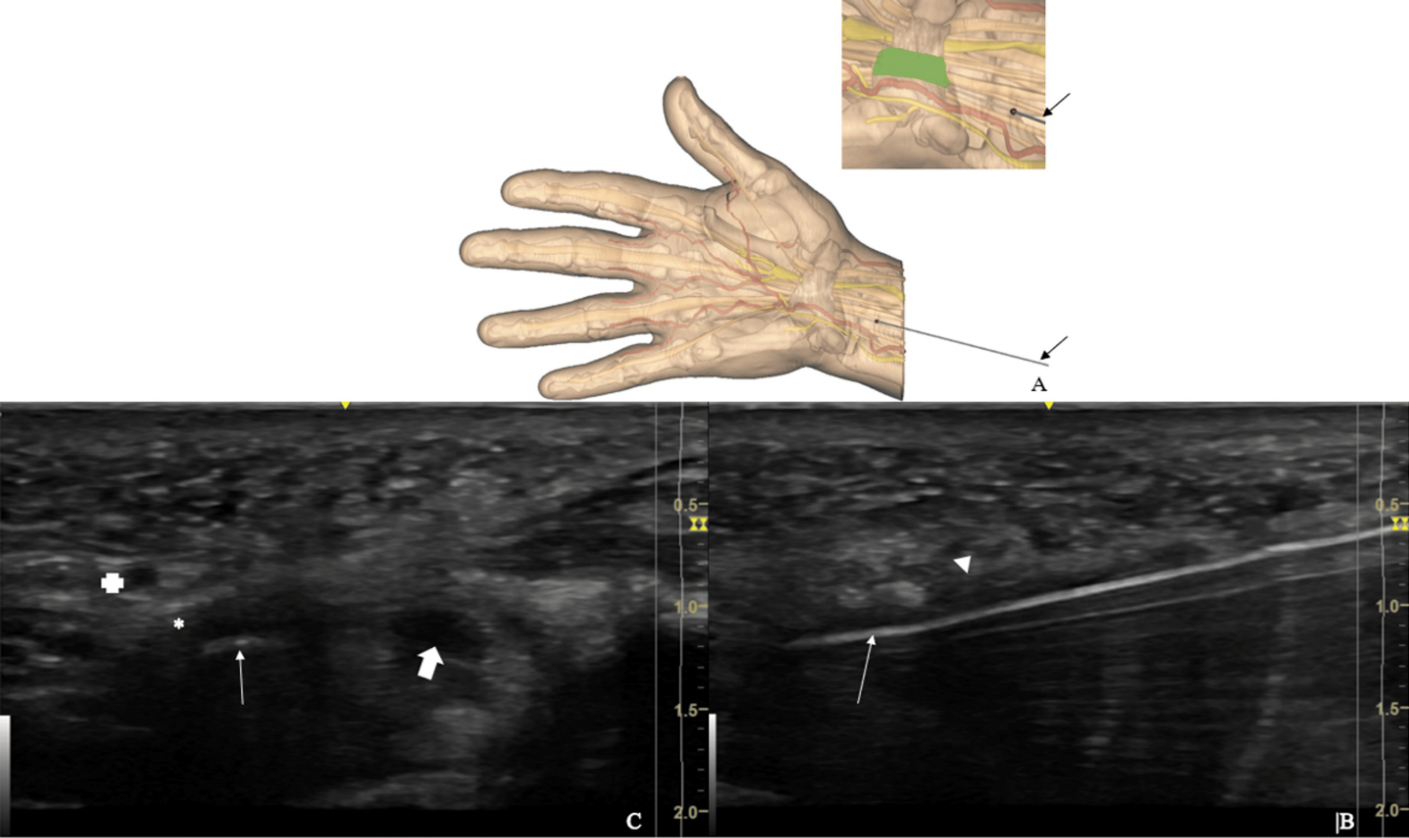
The round-ended stylus (arrow) is introduced through the entry point (red dot) in the carpal tunnel and defines the trajectory that the cutting instrument will follow in the safe zone between the hamate hook and the median nerve (green area) (A). Under sonography, the stylus (arrow) can be seen in the long-axis view under the TCL (arrowhead) (B). Short-axis view allows us to define the position of the future cut relative to the median nerve (solid arrow), the hamate hook (star) and the ulnar artery (cross) (C). This figure is the original work of the authors. TCL: Transverse carpal ligament
